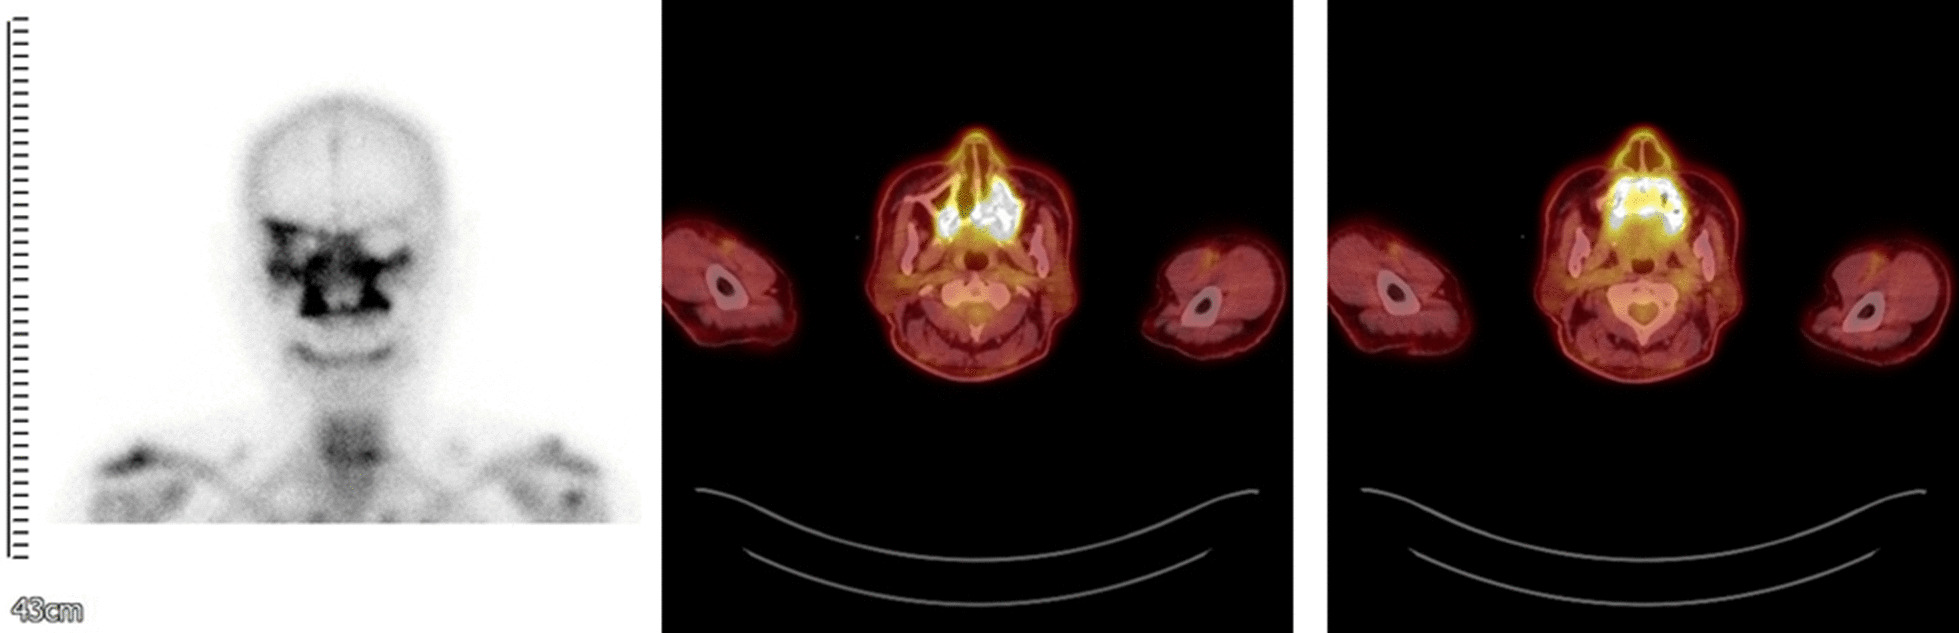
Bone scan showing active bone lesion around both maxillary and right whole skull base PET-CTs also showing irregular hypermetabolic lesion involving skull base, posterior nasopharyngeal wall, and both maxillary area
